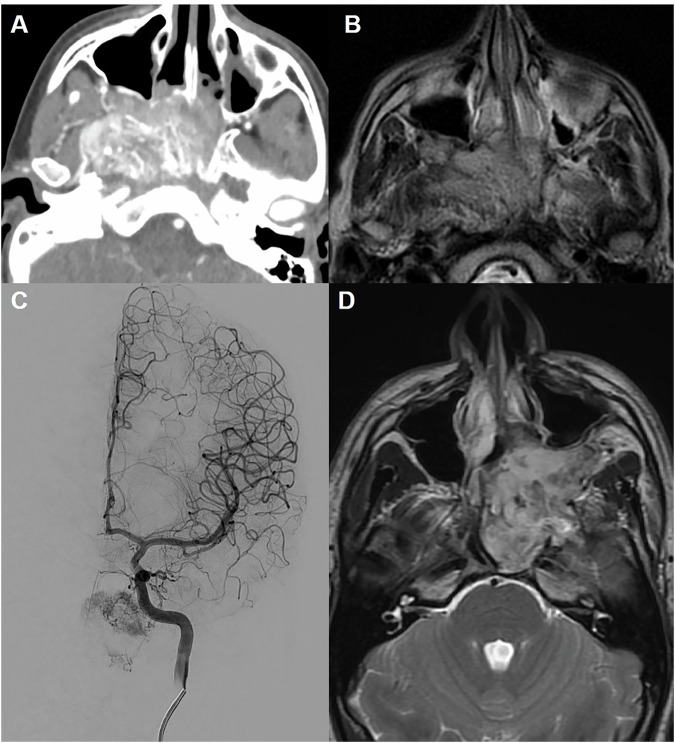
Juvenile nasopharyngeal angiofibroma (JNA). Axial CT angiogram (A), axial T2w MR image (B). A large, highly vascular soft tissue lesion is noted in the posterior choana and nasopharynx, compromising the nasopharyngeal airway. It extends to the left expanded and remodeled pterygopalatine fossa. From the pterygopalatine fossa, it extends through the pterygomaxillary fissure to the right infratemporal fossa and masticator space. Another JNA case: Digital subtraction angiogram (C) and axial T2w MR image (D). There is a sizeable arterial blush corresponding to the known left-sided JNA. There are arterial feeders arising from the ipsilateral internal carotid artery, the internal maxillary artery and the left ophthalmic artery. On MR, there is an inhomogeneous, well-circumscribed mass centered on the widened left sphenopalatine foramen with extension into the nasal cavity and nasopharynx. There is also widening of the left pterygomaxillary fissure with a little extension into the infratemporal fossa and masticator space
